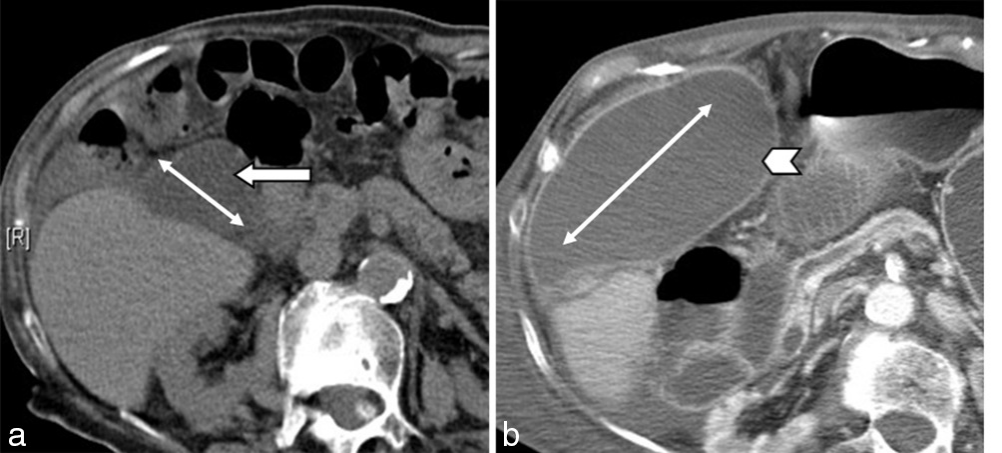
(a) Axial slice from a recent CT pulmonary angiogram used for comparison demonstrates a gallbladder normal in size and position (arrow). The axis of the gallbladder from neck to fundus is highlighted (double-sided white arrow). (b) There is a dramatic change in the anatomical position of the gallbladder (arrowhead) with a horizontal lie and grossly distended state—two signs of gallbladder volvulus. The striking change in the axis (double-ended white arrow) is significant.
